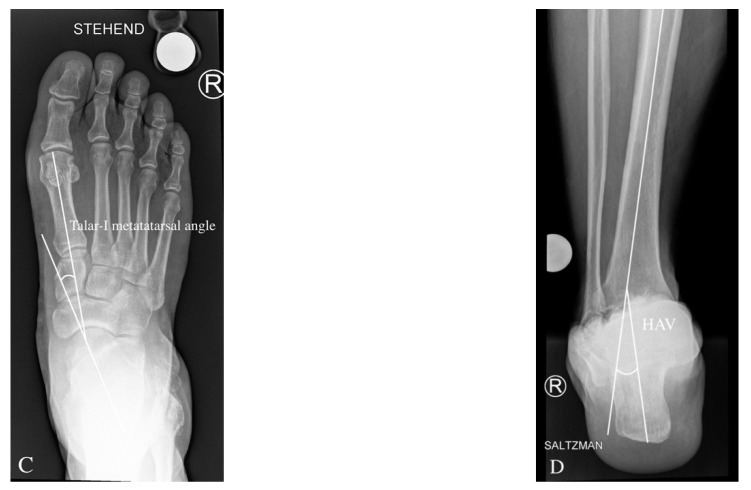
Angles for the Radiological Evaluation of a Varus Ankle Osteoarthritis. On the anteroposterior view, tibial anterior surface angle TAS (also known as medial distal tibial angle MDTA) tibiotalar surface (TTS) and talar tilt (TT) angle are shown (A). On lateral ankle radiograph, the tibial lateral surface angle (TLS) is assessed (B). The talar-1st metatarsal angle gives us a hint about the amount of midfoot deformity, both on lateral and dorsoplantar view (B,C). Inframalleolar deformity can be evaluated on the Saltzman view by measuring the hindfoot alignment view (HAV) angle (D).
